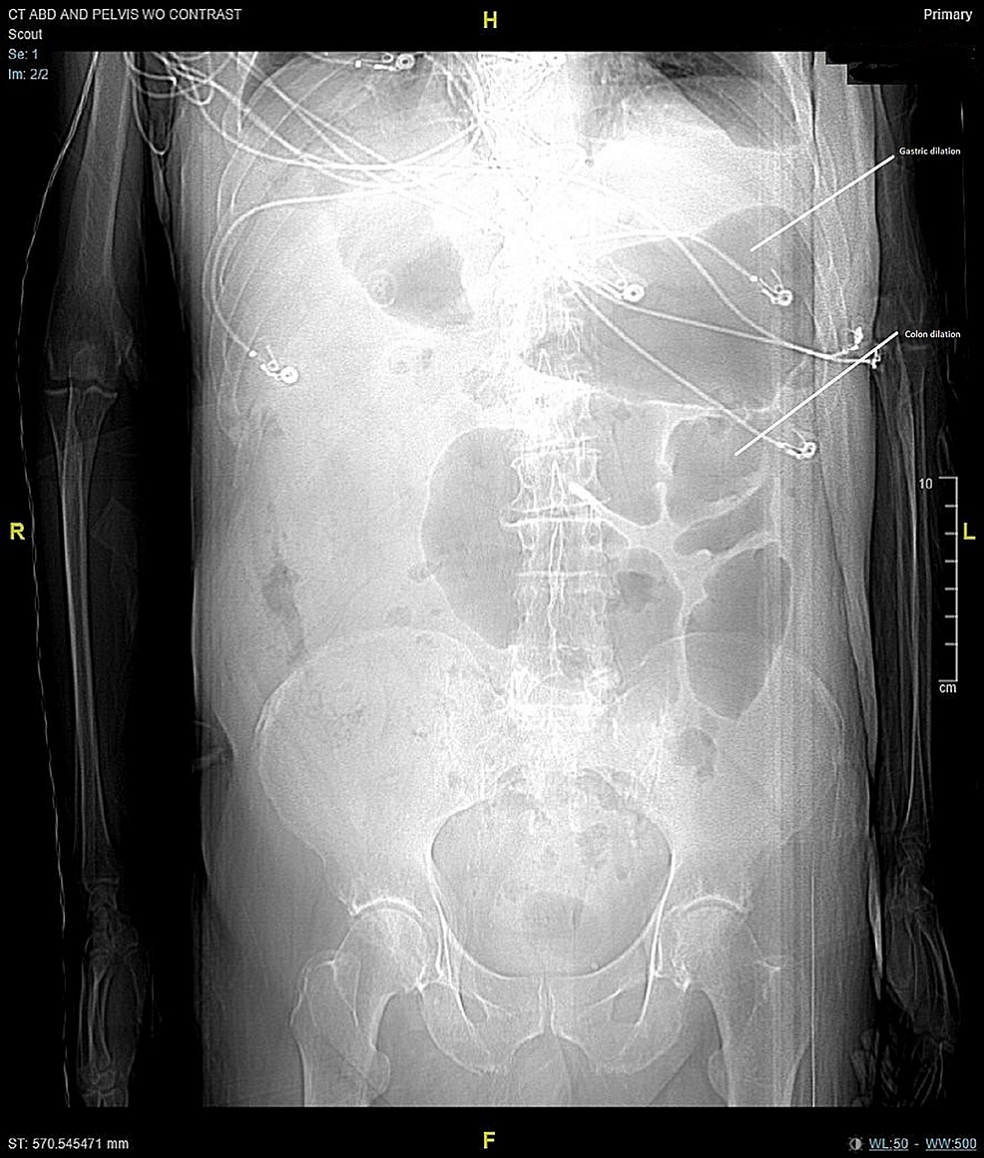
CT of the abdomen/pelvis on worsening of infection. Scout: Large bowel dilation. CT: computed tomography
